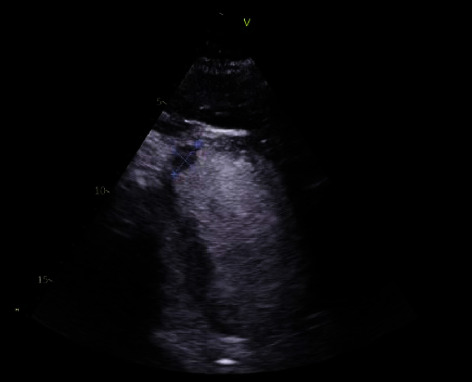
Contrast-enhanced transthoracic echocardiogram. Contrast ECHO demonstrating the left ventricular thrombus.
